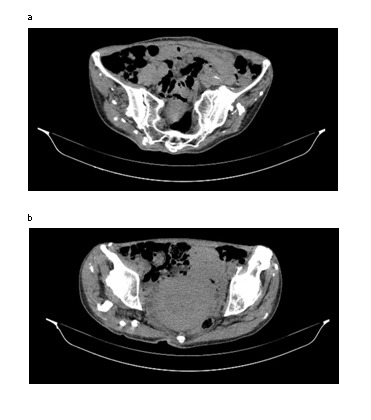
CT aspect. Calcifications of the gluteal muscles and at the trochanterian insertion of the left psoas muscle
